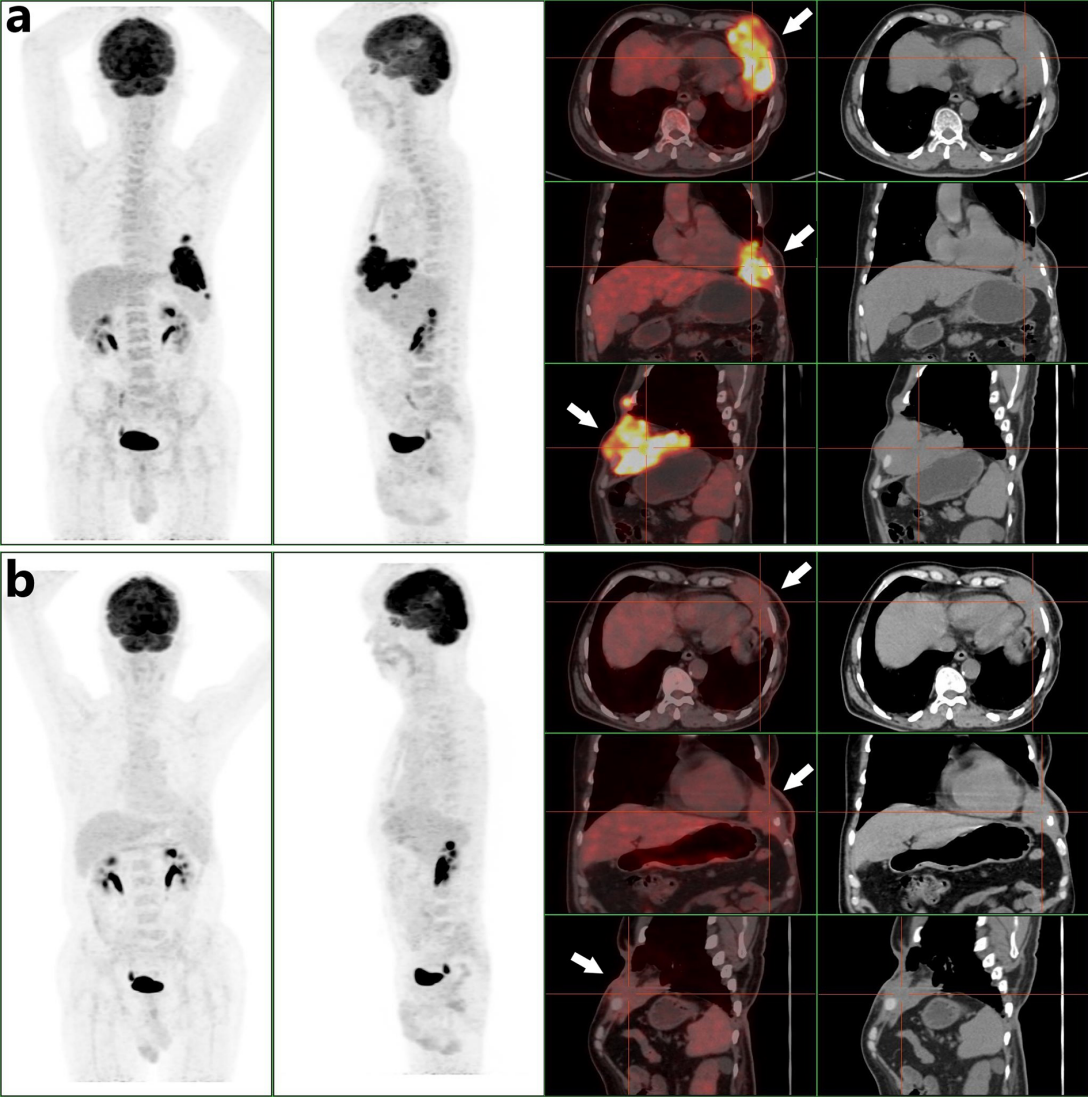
(A) A PET/CT scan obtained before combined oncotherapy on April 9 2019 (white arrows, the tumor mass with high FDG metabolism). (B) A PET/CT scan obtained before surgery on September 30 2019 (white arrows, the tumor mass was smaller than before and its FDG metabolism was less than before).
